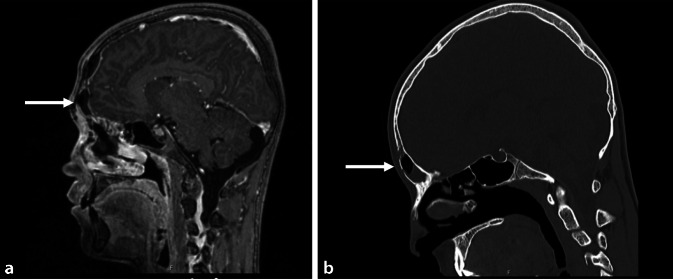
Pott’s puffy tumor with a connection to the frontal sinus of the left side (*arrows*) (a MRI-, b CT-scan, sagittal)
